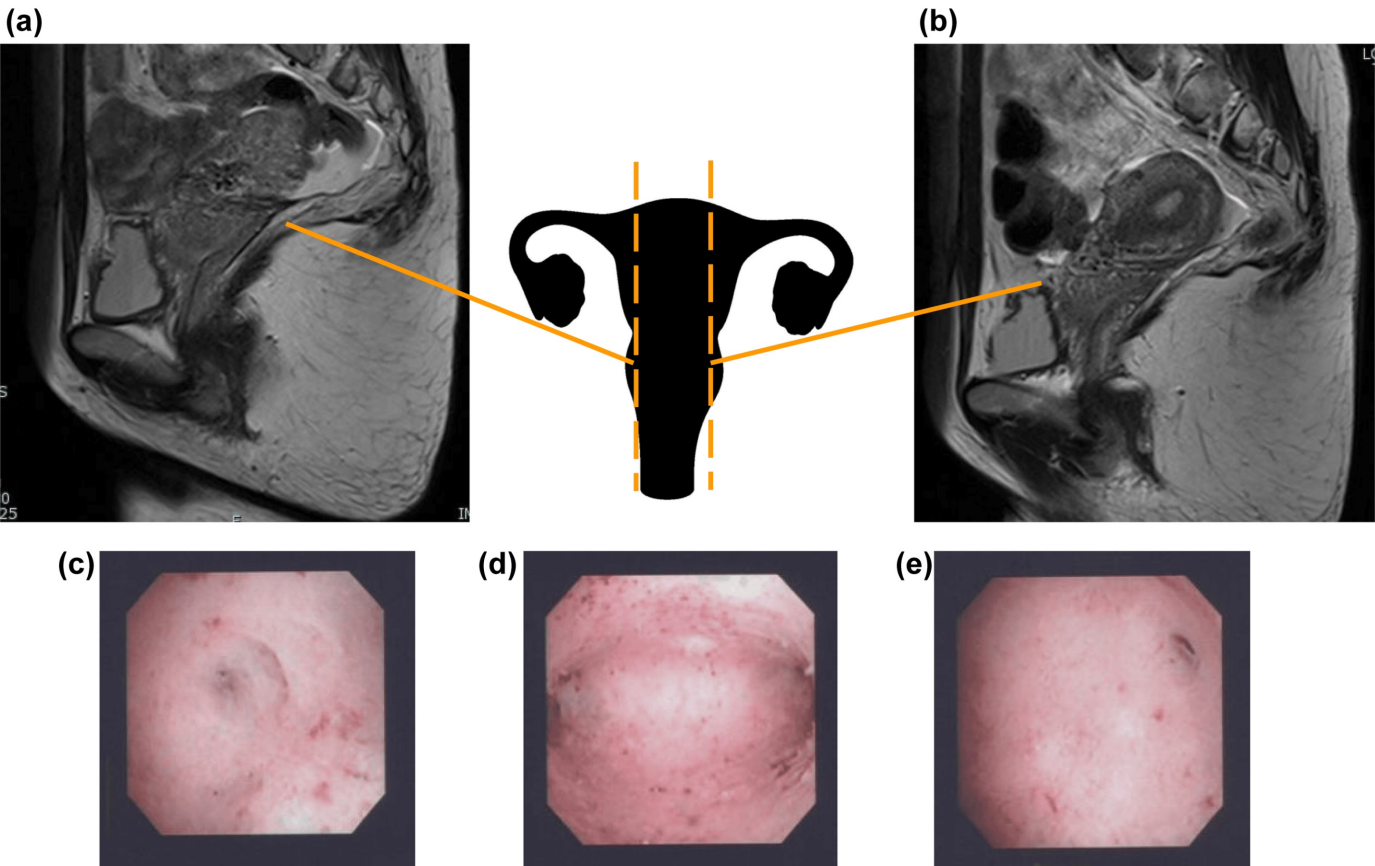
Evaluation of non-absorbable sutures using non-invasive methods. (a, b) T2-weighted MRI images six months postoperatively. Low-signal images indicating hemosiderin deposition were observed at the locations where the compression sutures had been placed; however, the sutures were not visible. (c-e) Hysteroscopic images taken one year postoperatively. (c) No obvious abnormal findings were observed in the area around the right fallopian tube opening. (d) No adhesions or sutures were observed in the uterine cavity. (e) No obvious abnormalities were observed in the area around the left fallopian tube opening.
